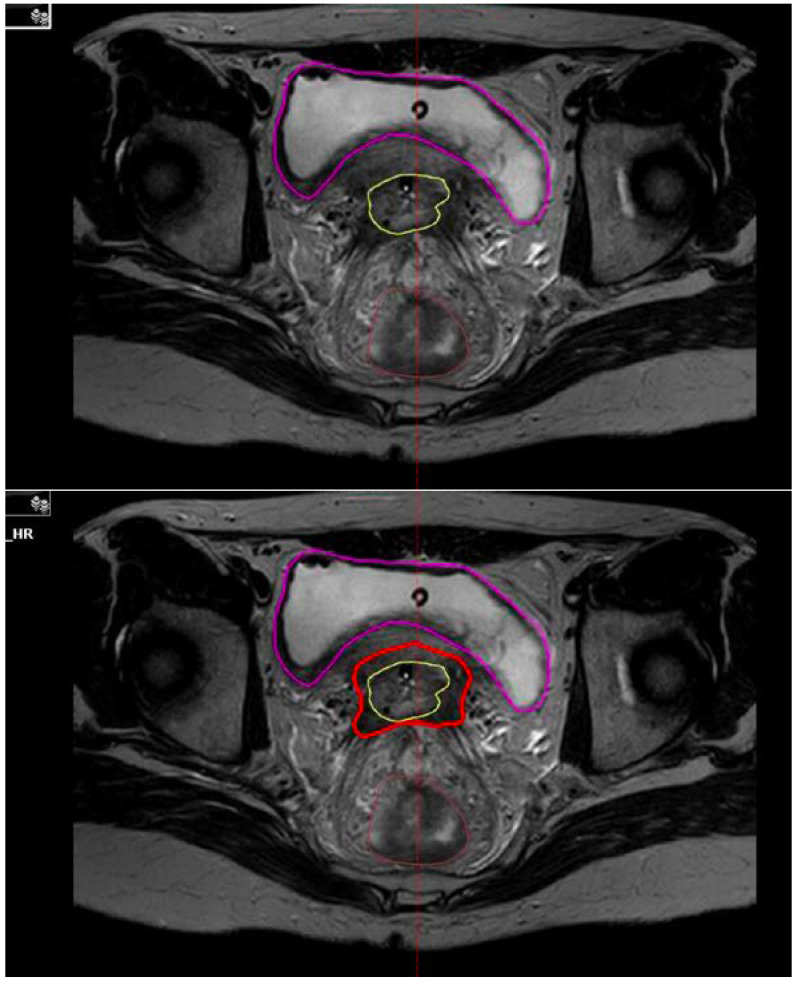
Residual gross tumour volume at brachytherapy (GTV-Tres: yellow) and adaptive High Risk CTV-T (CTV-T HRadapt: red) contoured at the time of brachytherapy on axial T2 MRI.
